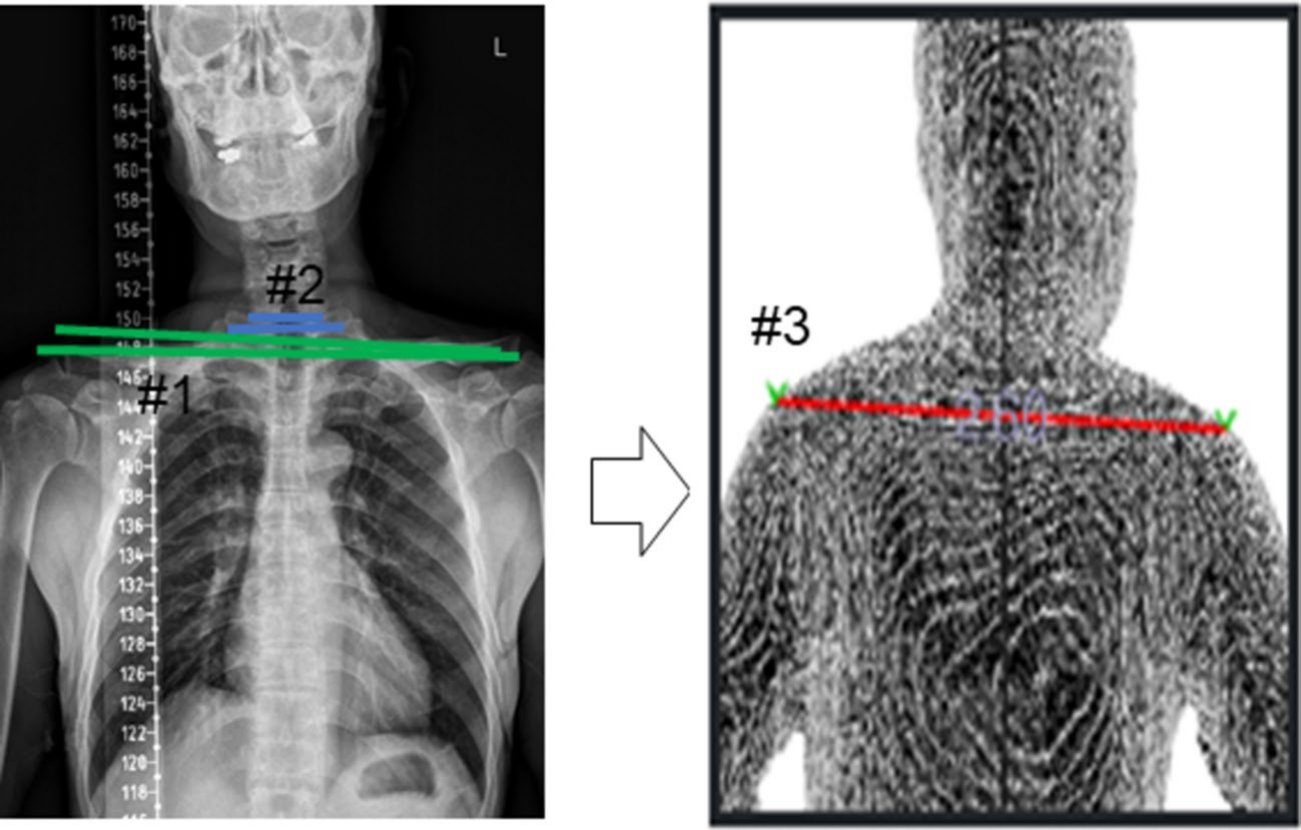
#1. Clavicle angle / #2. T1 coronal tilt on x-ray / #3. Shoulder gradient on the 3D scanner
